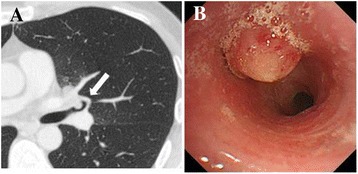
Chest computed tomography and bronchoscopy findings. a Computed tomography scan performed five months after starting cART showing the endobronchial mass (arrow). b Bronchoscopy showing the endobronchial mass in the left upper lobe bronchus
